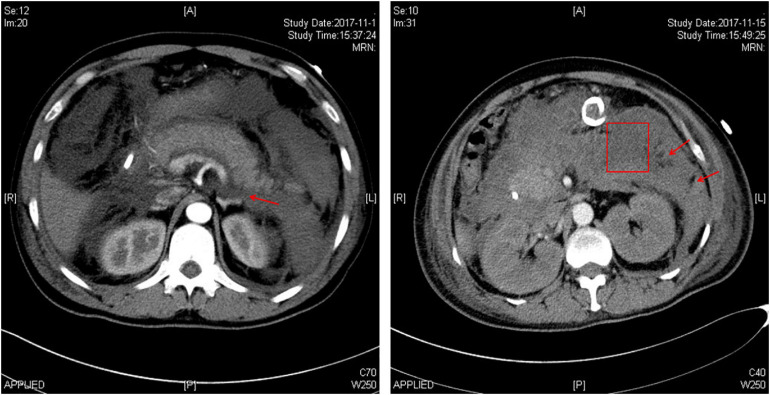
Abdominal computed tomography images of the patient showing the affected pancreas. The left image was taken on the third day of onset of acute pancreatitis, showing peripancreatic exudation (arrow) and the enlarged pancreas with adjacent water density shadow. The right image was taken on the eighteenth day of disease onset, showing the occurrence of infected pancreatic necrosis. The box indicates a partial necrotic pancreas whilst the arrows indicate infective necrosis.
